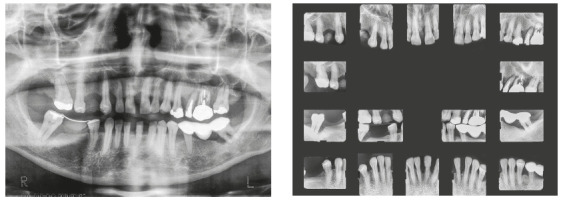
Initial radiographs of Case 3.
